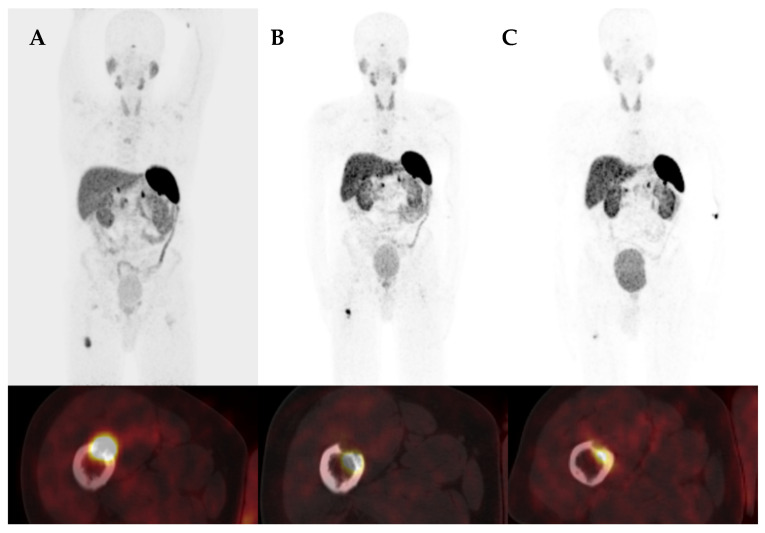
[^68^Ga]Ga-DOTATATE PET/CT images of a 44-year-old man with a near 4-year history of osteoporosis and hypophosphataemia with multiple bone fractures (Case 1). The [^68^Ga]Ga-DOTATATE PET/CT images showed intense focal uptake in the right femur with an SUVmax of 14.2 (A). The follow-up [^68^Ga]Ga-DOTATATE PET/CT revealed focal recurrence with an SUVmax of 14.3 (B). Thermoablation was the next step, but phosphate was still below the normal level. The subsequent [^68^Ga]Ga-DOTATATE PET/CT revealed a focal recurrence with an SUVmax of 7.9 (C). Upper row—MIP images, bottom row—fused transaxial PET/CT images of the right femur.
